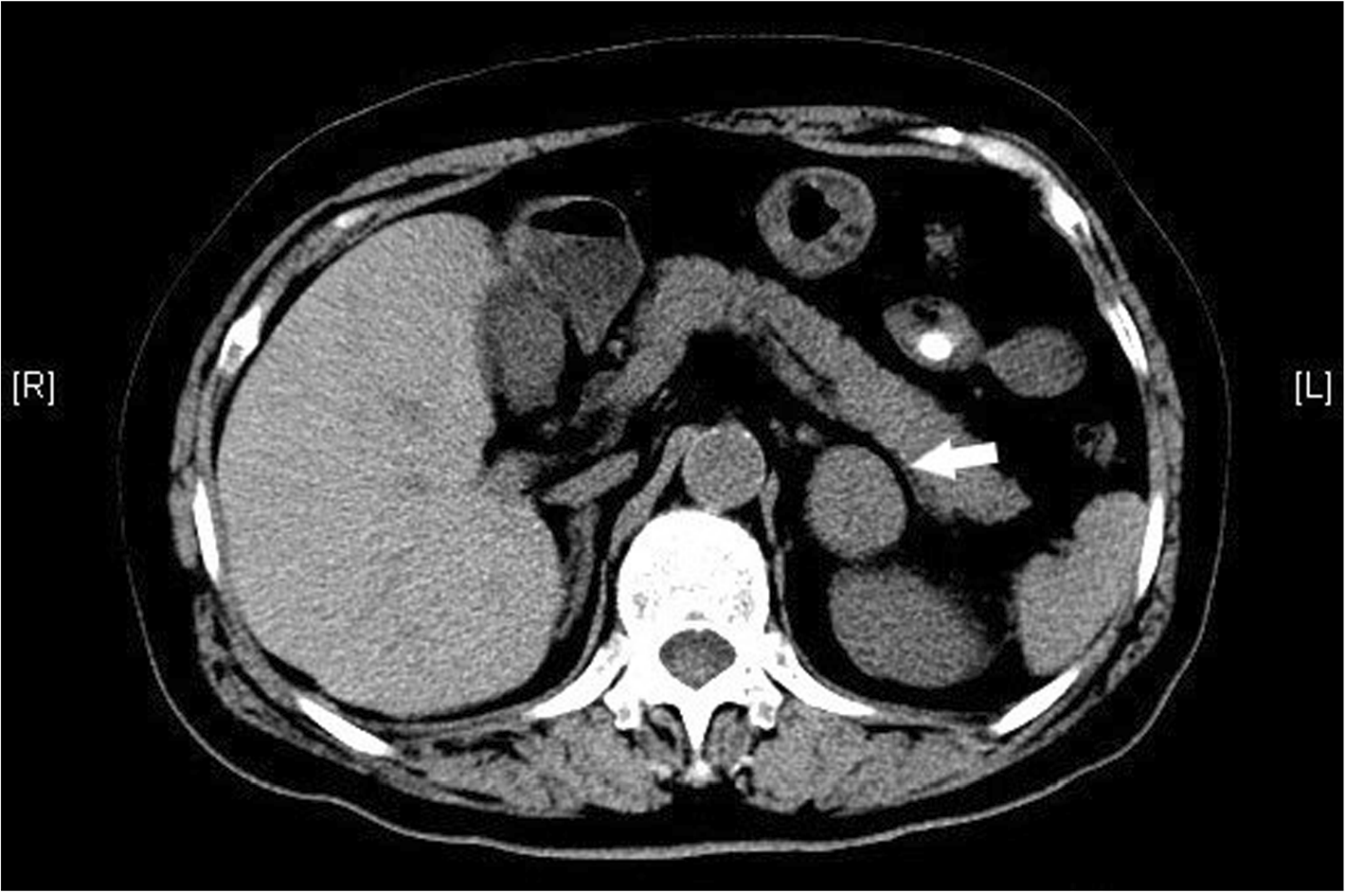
Abdominal computed tomography image of a 4.5 cm × 3.1 cm solid left adrenal mass (*arrow*)
